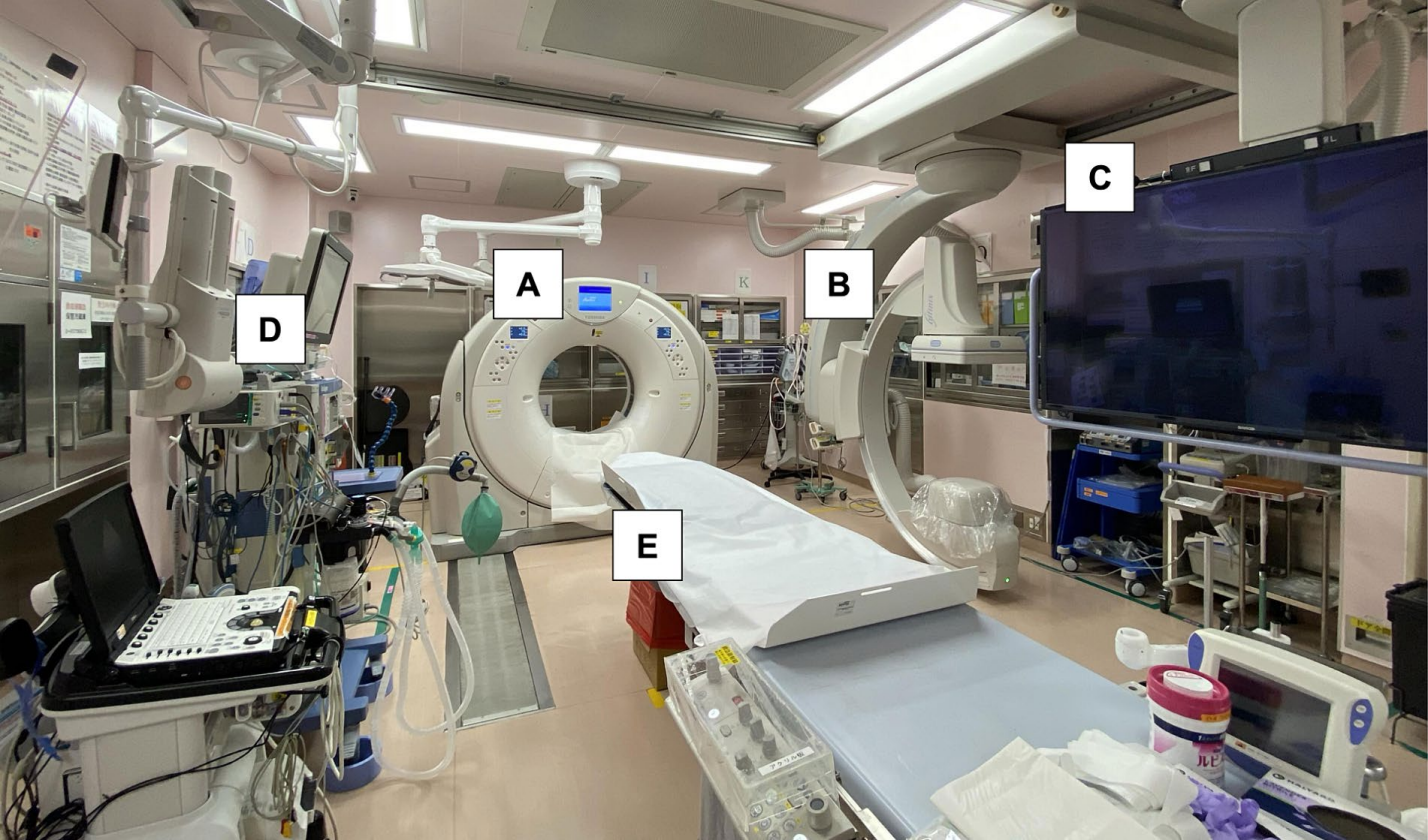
Photograph showing a sliding computed tomography scanner system with interventional radiology features in the emergency room. Acute procedures for ischemic stroke, including airway management, and angiography can be performed on the table without transferring the patient. (A) Sliding computed tomography (CT) scan device, (B) movable C-arm, (C) monitoring screen, (D) mechanical ventilator with anesthesia apparatus, and (E) CT examination and intervention table.
